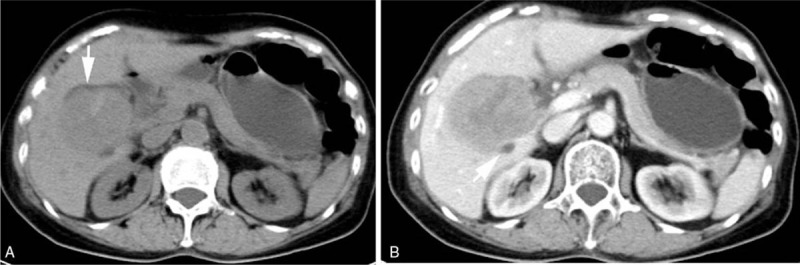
Computed tomography imaging revealed a circular low-density shadow of approximately 7.5 × 8.0 cm in the hepatic hilar region. The interior of the tumor was uneven, and the strip demonstrated high density. (A) The tumor was unevenly enhanced during scanning of the portal venous phase, and the internal density was uneven. (B) The mass compressed the intrahepatic bile duct and caused dilatation (arrow).
